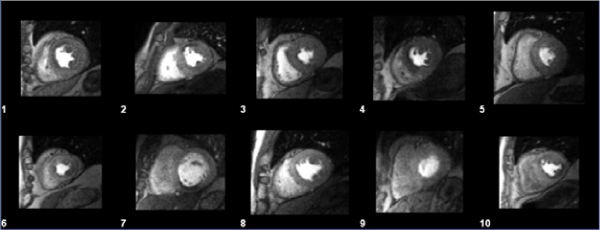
Representative dynamic frames from ten accelerated DCE-MRI datasets.
